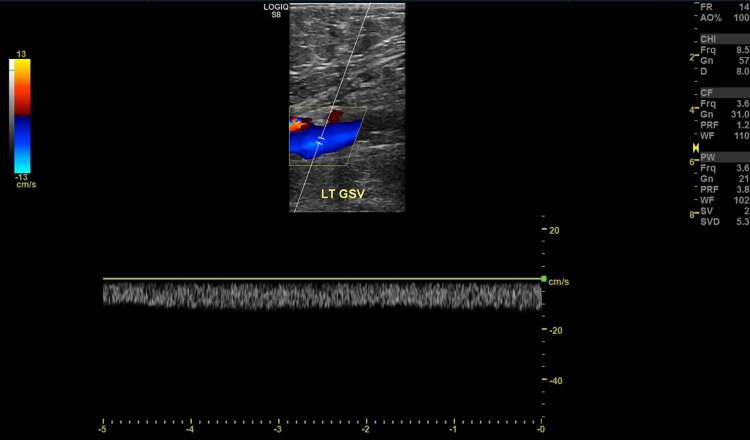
Doppler waveforms and partial occlusion within the left great saphenous vein.
